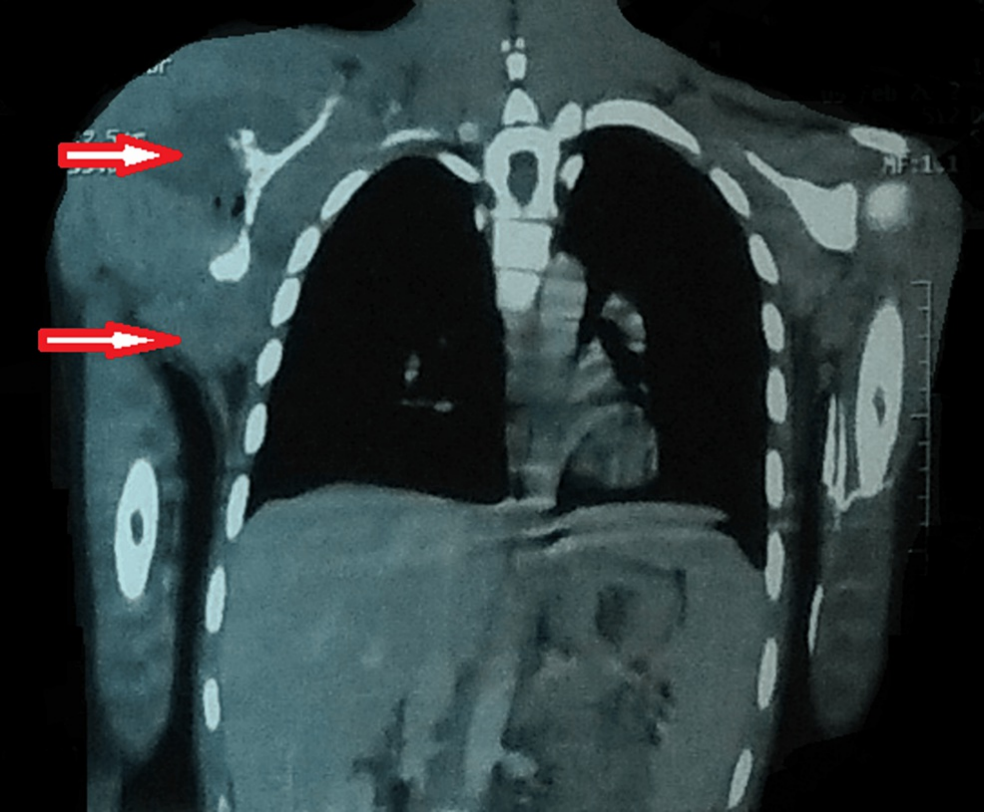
A CT of the chest showing a soft tissue density lesion. Multiple air foci were noted involving the right scapula, with soft tissue extension into the right axillary region involving the subscapular, supraspinatus, and infraspinatus fossa. It showed a soft tissue extension into the glenoid cavity and involved the right posterolateral chest wall CT: computed tomography
